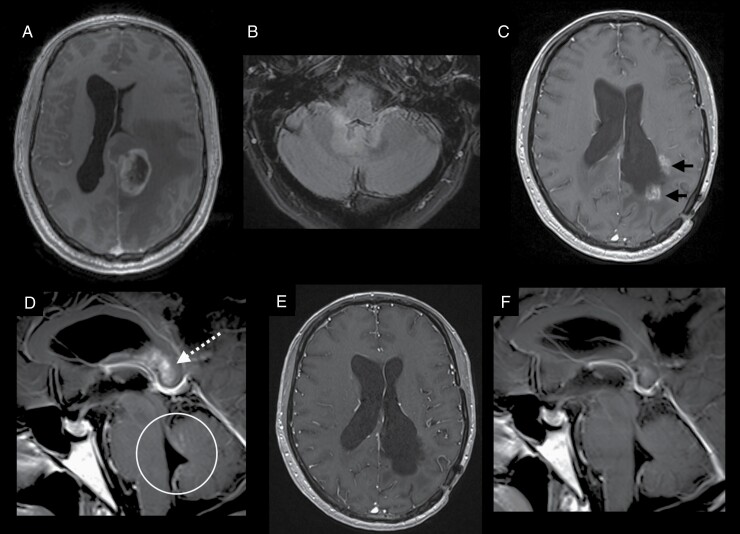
MRI brain images. (A) Axial T1 post-contrast image at the time of diagnosis showed an irregular rim enhancing lesion in the left fronto-parietal lobe with marked surrounding vasogenic edema. (B) Axial T2 FLAIR post-contrast image 22 months later showed new FLAIR hyperintense lesion around the fourth ventricle, suspicious for tumor recurrence. (C) Axial and (D) sagittal T1 post-contrast images (37 months from the time of diagnosis) showed enhancing lesions around the surgical cavity (black arrows), extending into the splenium of the corpus callosum (white dotted arrow). Leptomeningeal and ependymal enhancements were also observed within the posterior fossa, centered around the fourth ventricle (white circle). (E,F) Follow-up MRI brain 3 months after the initiation of intravenous bevacizumab showed near complete resolution of the previously seen enhancing lesions.
